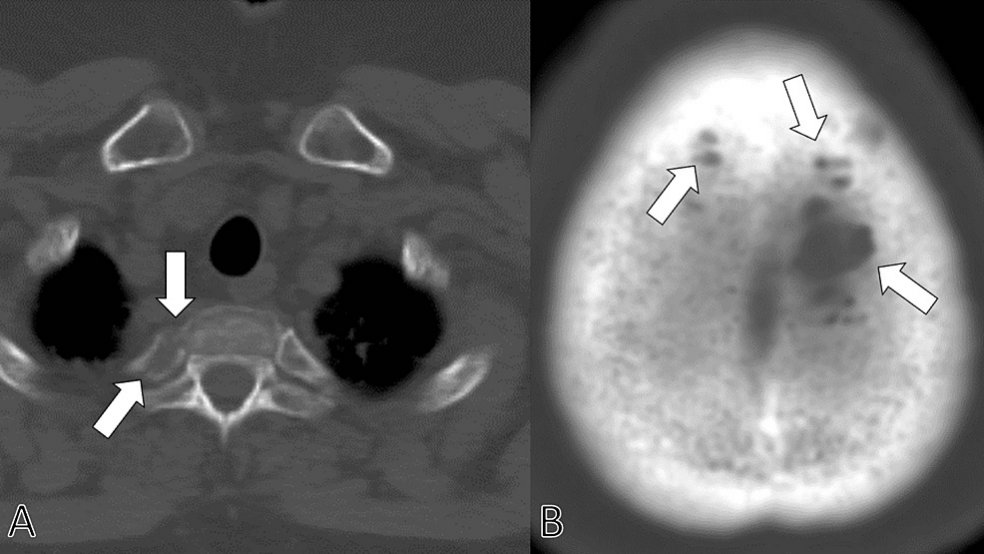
Systemic computed tomography clarifying multiple bone lytic lesions on the ribs (A) and skull (B) (arrows).
